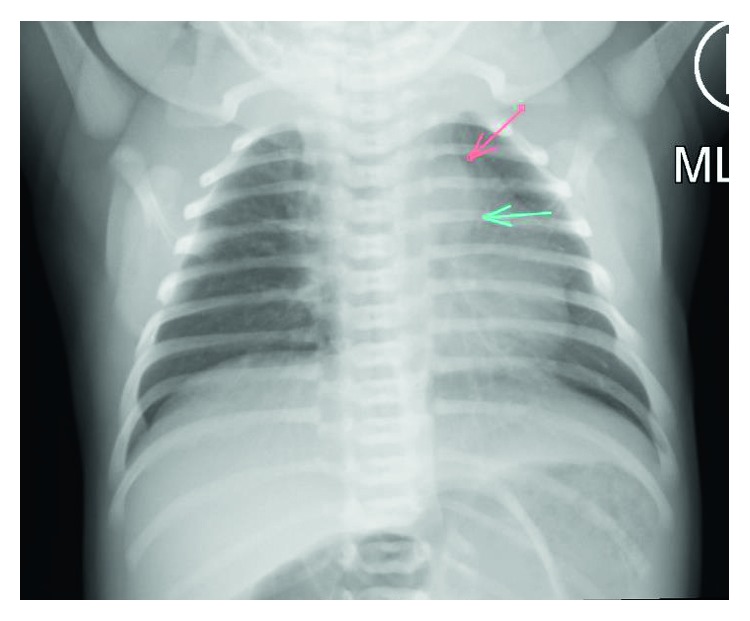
Chest radiograph at presentation: arrows indicate lucency of the ductus arteriosus aneurysm.
